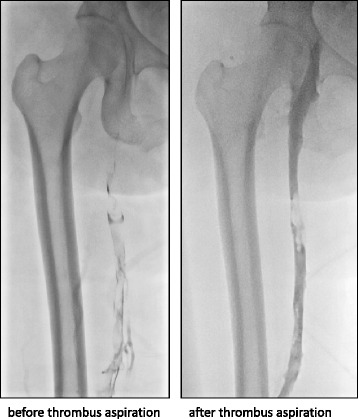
Representative images of X-ray venography before and after thrombus aspiration. X-ray venography shows an extensive filling defect before thrombus aspiration along the femoral vein, and a considerable reduction in the defect after thrombus aspiration
